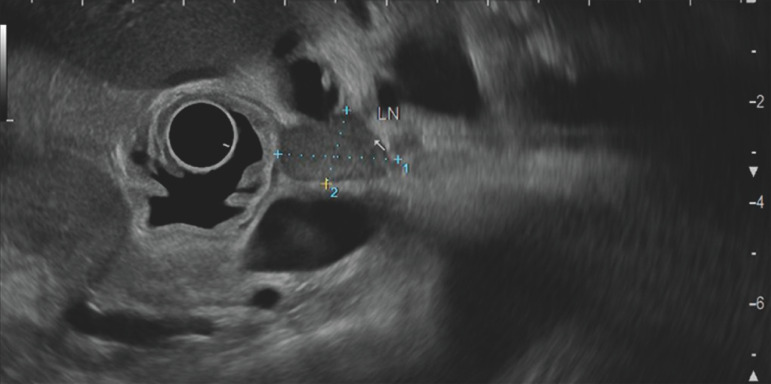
Endoscopic ultrasound image showing dilatation of the biliary tract with hypoechogenic irregular thickening of the distal common bile duct.
